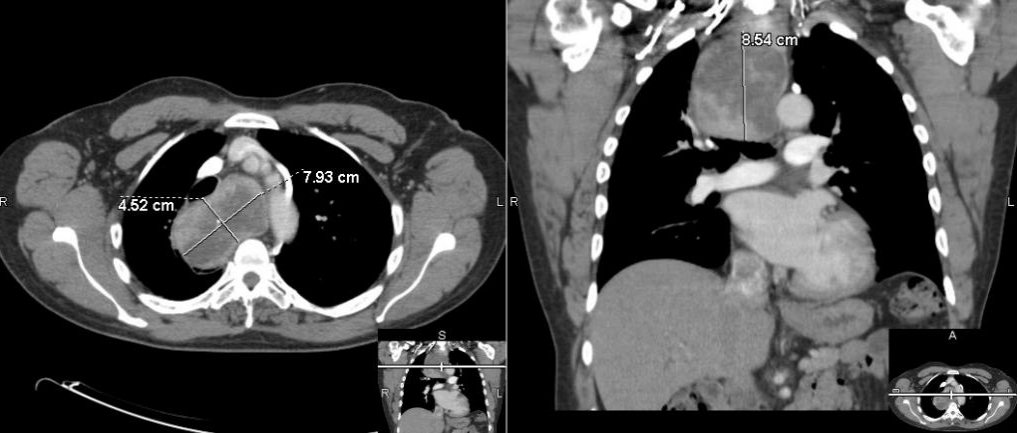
CT of the chest revealing a posterior mediastinal mass that extends all the way down to the aortic arch with tracheal compression and deviation.
